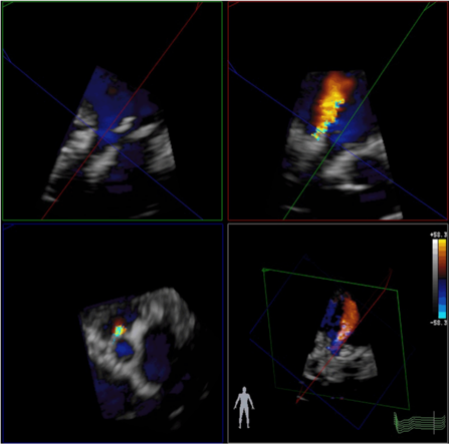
Assessment of the neck of PVL with three dimensional echocardiographyNewly developed I-Rotate transducers do allow a full electronic rotation of 360° (adjustable by 5° steps) around the CoreValve prosthesis with excellent spatial and temporal resolution [21]. Similarly as studying the PVL extent of a mitral prosthesis with transesophageal echocardiography it may now be possible to study the PVL extent of a TAVI prosthesis by use of I-rotate colour Doppler in apical views, so avoiding interference with imaging of PVL jets by crushed native material or the stent (Fig. 16).
